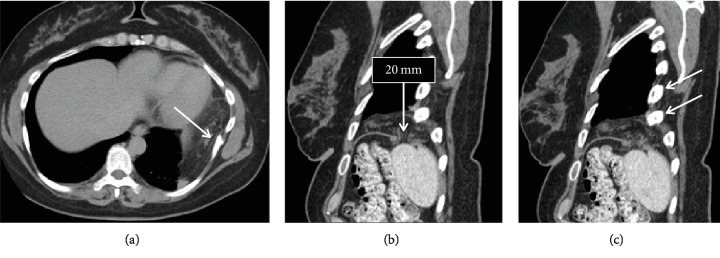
Axial (a) and sagittal (b and c) CT scan images showing diaphragmatic omental herniation. (a) also shows a healed rib fracture (arrow), which is adjacent to the herniated omentum. Two healed rib fractures are also indicated by arrows in (c).
